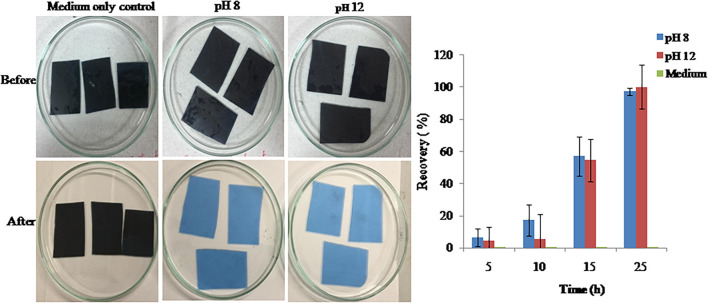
Hydrolysis of gelatin layer from used X-ray films by PyCP.
